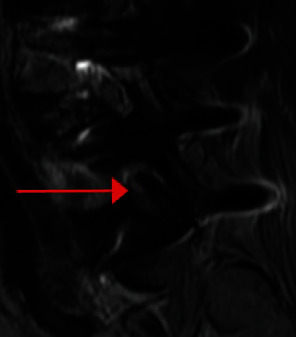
Postoperative sagittal T2 MRI demonstrating persistent L5 nerve compression in the L5-S1 foramen (arrow).
